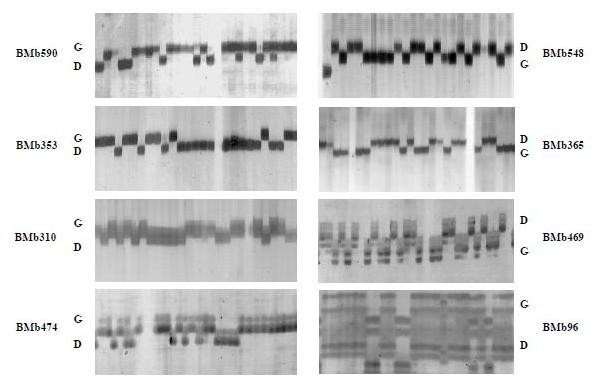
Representative BMb markers. BES-SSR markers scored in a subset of recombinant inbred lines of the mapping population based on the cross DOR364 (D) × G19833 (G). Markers BMb590, BMb548, BMb353 and BMb365 have AT motifs, BMb310 has an ATA motif and BMb469, BMb474 and BMb96 represent the motifs AGA, CT and CA, respectively.
